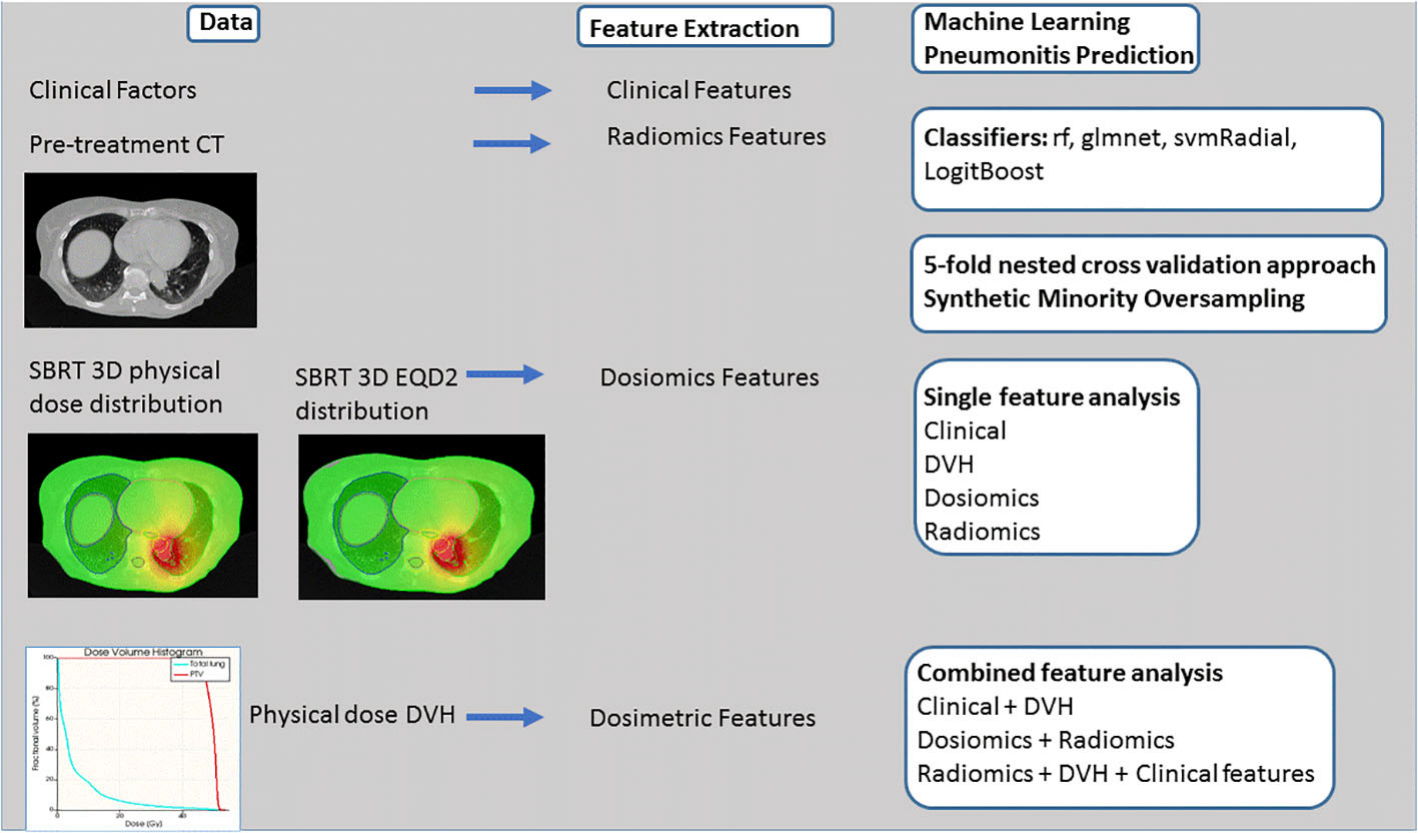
Process flow. Clinical, Computed Tomography (CT) and 3D dose volume and dose volume histogram (DVH) data is used for feature extraction. PTP prediction is performed testing different classifiers such as random forest (rf), logistic elastic net regression (glmnet), support vector machine (svmRadial), and logitBoost and 5-fold nested cross validation approach and Synthetic Minority Oversampling. Four single models and five combined models are analyzed.
